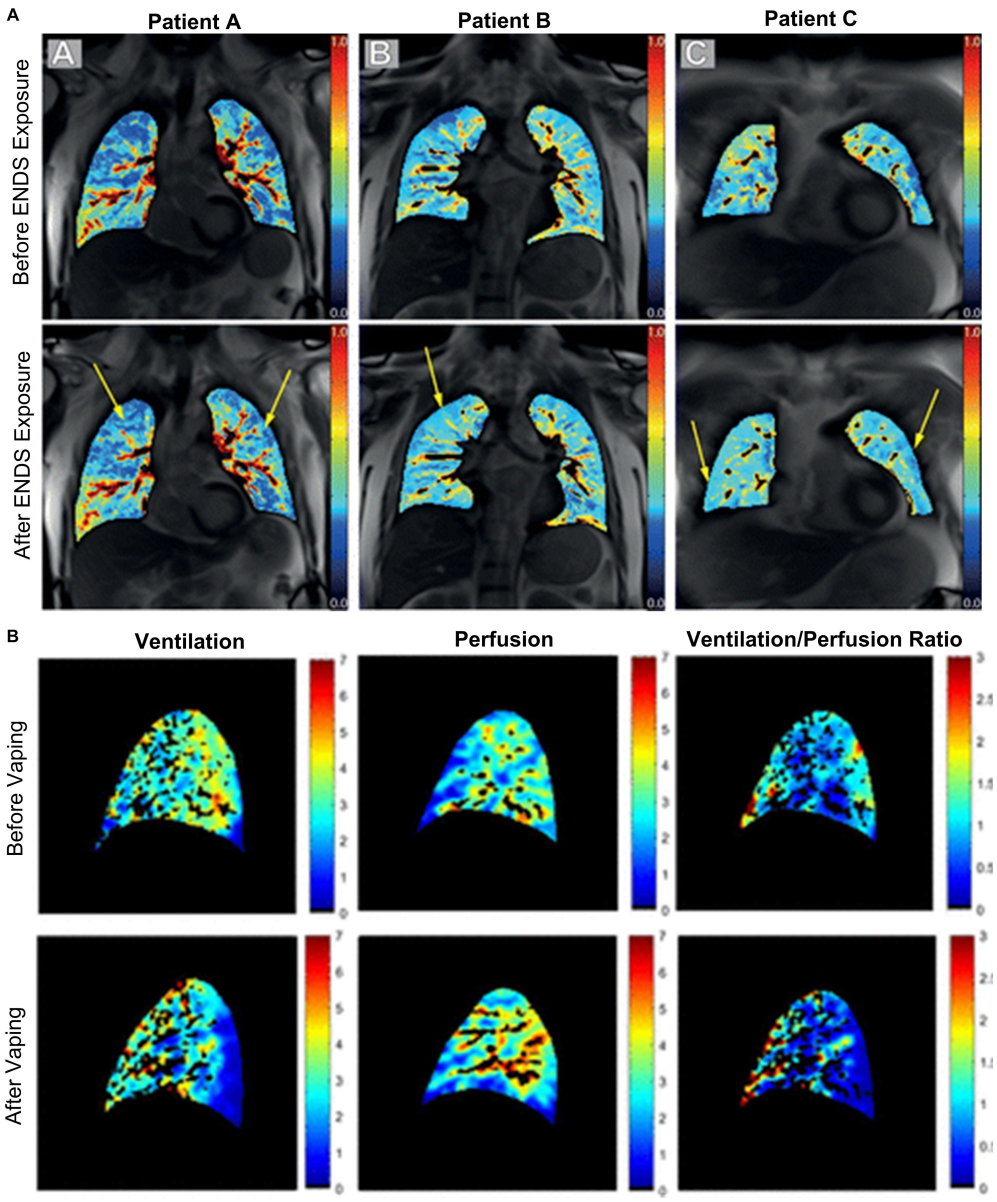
Free-breathing ^1^H MRI of acute effects of vaping. (A) Pulmonary perfusion images obtained by using non-contrast matrix pencil MRI in three electronic nicotine vape users before and after exposure. The arrows indicate lung regions with increased regional perfusion post-exposure. Red corresponds to greater perfusion amplitude and blue corresponds to lower values (108). (B) Spatial maps of ventilation, perfusion, and ventilation-perfusion ratios, before and after vaping, in a single sagittal slice of one representative subject. Black regions within the lung field represent regions excluded from analysis, including conducting airways and vessels, and regions with poor signal to noise (107).
